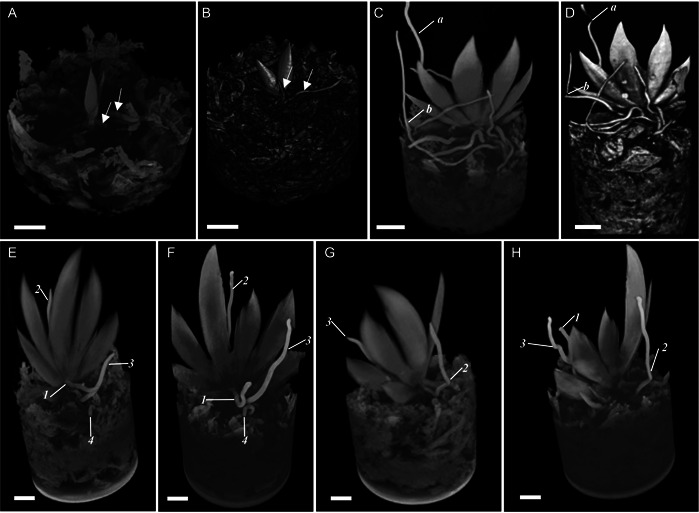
Screen recordings of two sides of a Falcon container containing a seedling (A, B), flowering plant (C, D), and non‐flowering plant (E–H) of *Erycina pusilla* during repeated micro–computed tomography (CT) scanning over a 12‐week period. (A, B) Scans of seedling roots (indicated with white arrows) that resupinated within a four‐day period, shown before (A) and after (B) resupination. (C, D) Scans of the roots of a flowering plant (indicated with “a” and “b”) that resupinated over a 12‐week period, shown before (C) and after (D) resupination. (E–H) Scans of the roots of a non‐flowering plant (indicated with 1, 2, 3, and 4) that resupinated over a 12‐week period. Panels E and F display scans of the front of a non‐flowering plant showing the roots before (E) and after (F) resupination; panels G and H show scans of the back of a non‐flowering plant before (G) and after (H) resupination. Scale bar = 1 cm.
